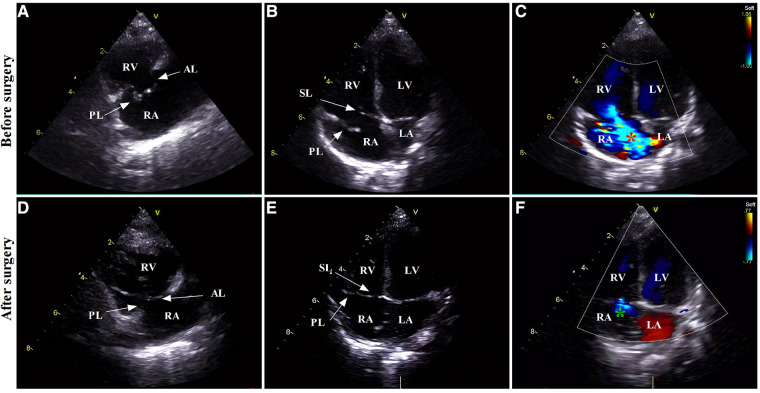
The transthoracic echocardiographic images before and after surgical repair. Preoperative RV inflow (A) and apical four-chamber views (B,C) showing dilation of RA/RV, systolic prolapse of AL/PL toward the RA (white arrows), and severe TR with right-to-left shunting through PFO (red asterisk). Postoperative echocardiogram (D–F) showing normalized RA/RV, complete edge-to-edge leaflet coaptation (white arrows), and trivial TR (green asterisk). RV, right ventricle; RA, right atrium; LA, left atrium; LV, left ventricle; AL, anterior leaflet; PL, posterior leaflet; SL, septal leaflet; TR, tricuspid regurgitation; PFO, patent foramen ovale.
